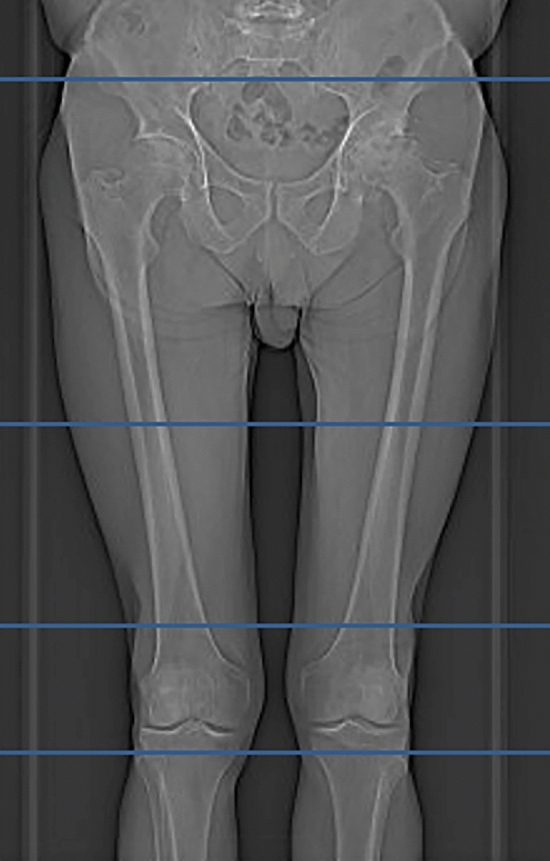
Image depicting the scout of the CT scan protocol. The scanned regions are bordered by the blue boxes and include the hip joint the proximal femur and the distal femoral condyle
